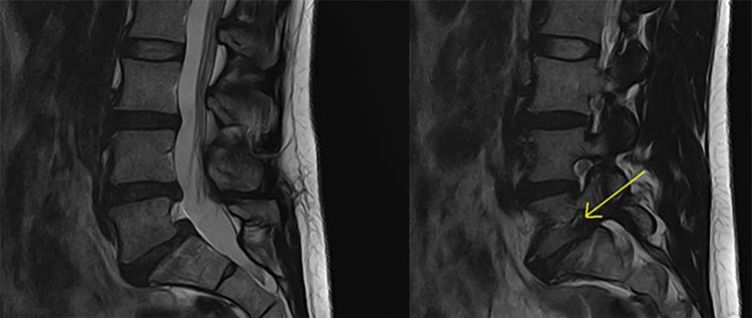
Preoperative MRI lumbar spine (mid- and para-sagittal slices of T2 weighted sequence) demonstrating posterior bulging of the L4/5 and L5/S1 discs in conjunction with a Grade 1 spondylolisthesis of L5 on S1 resulting in significant right-sided foraminal stenosis at L5/S1 (indicated by yellow arrow).
